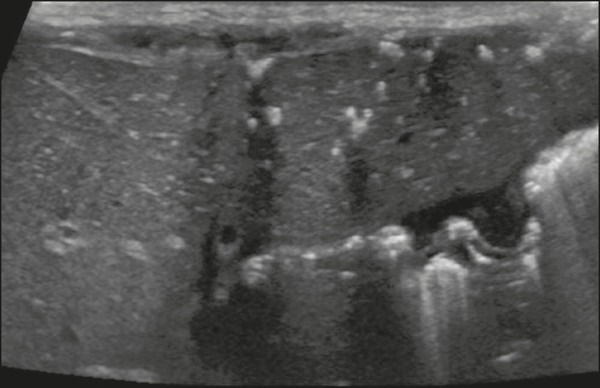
Biliary hamartomas in an otherwise healthy 2-month-old boy. Abdominal ultrasound showing multiple sparse hyperechoic foci along the biliary tract. Note that some foci produce the “comet tail” artifact, characteristic of cholesterol crystals, whereas others produce posterior acoustic shadowing, possibly corresponding to calcifications.
